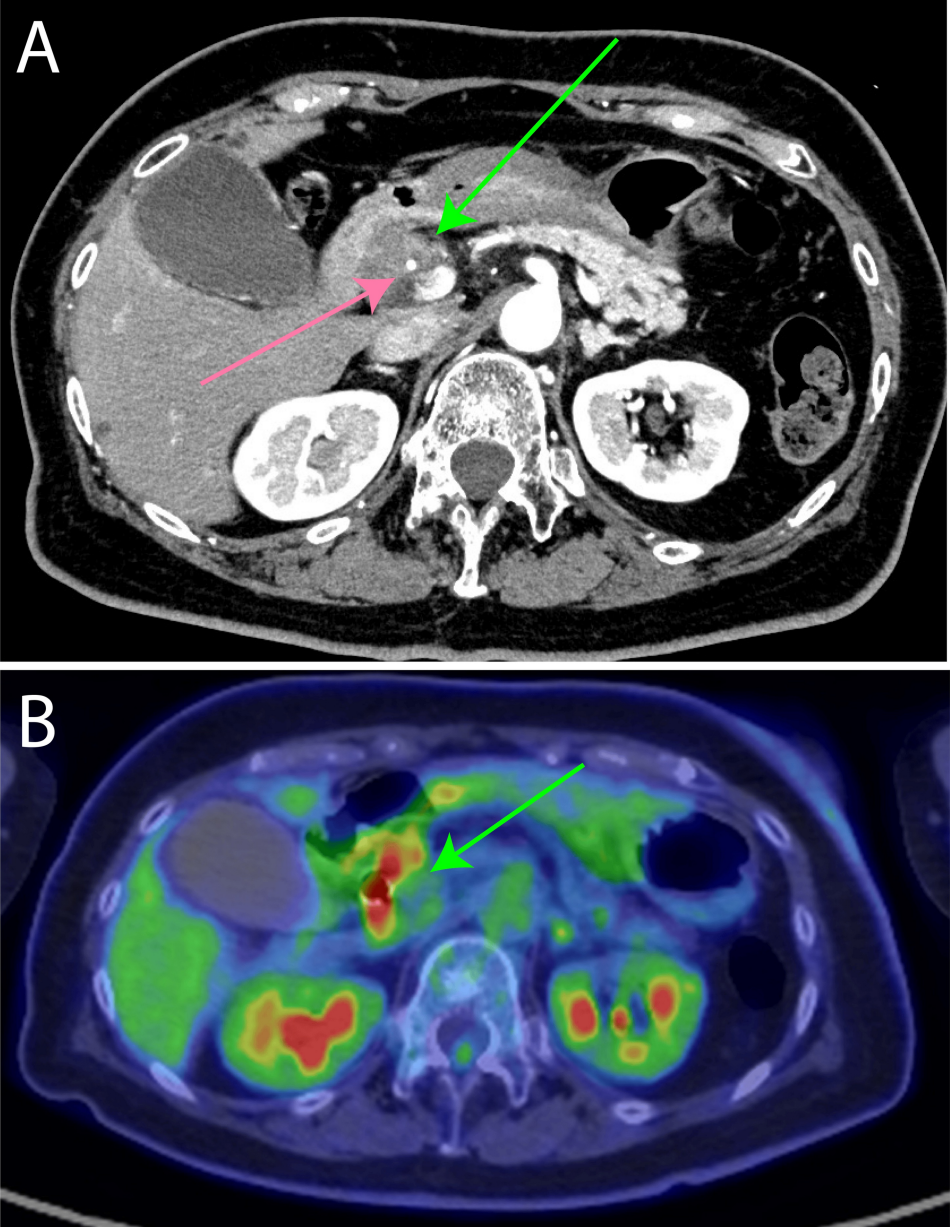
Images at the time of diagnosis of pancreatic cancer (patient's age: 72 years) (A) Contrast-enhanced CT showing a pancreatic head tumor (green arrow) with the gastroduodenal artery identified within the tumor (pink arrow); (B) Positron Emission Tomography-Computed Tomography (PET-CT) demonstrating radiotracer uptake corresponding to the tumor (green arrow).
